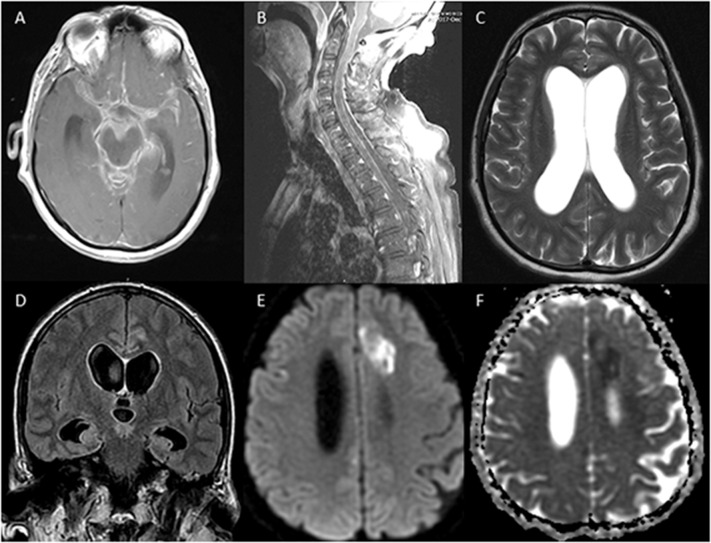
MRI of the brain and of the spine showing evidence of leptomeningeal enhancement (A,B), hydrocephalus (C,D), and acute infarction in the anterior cerebral artery territory (E,F).
